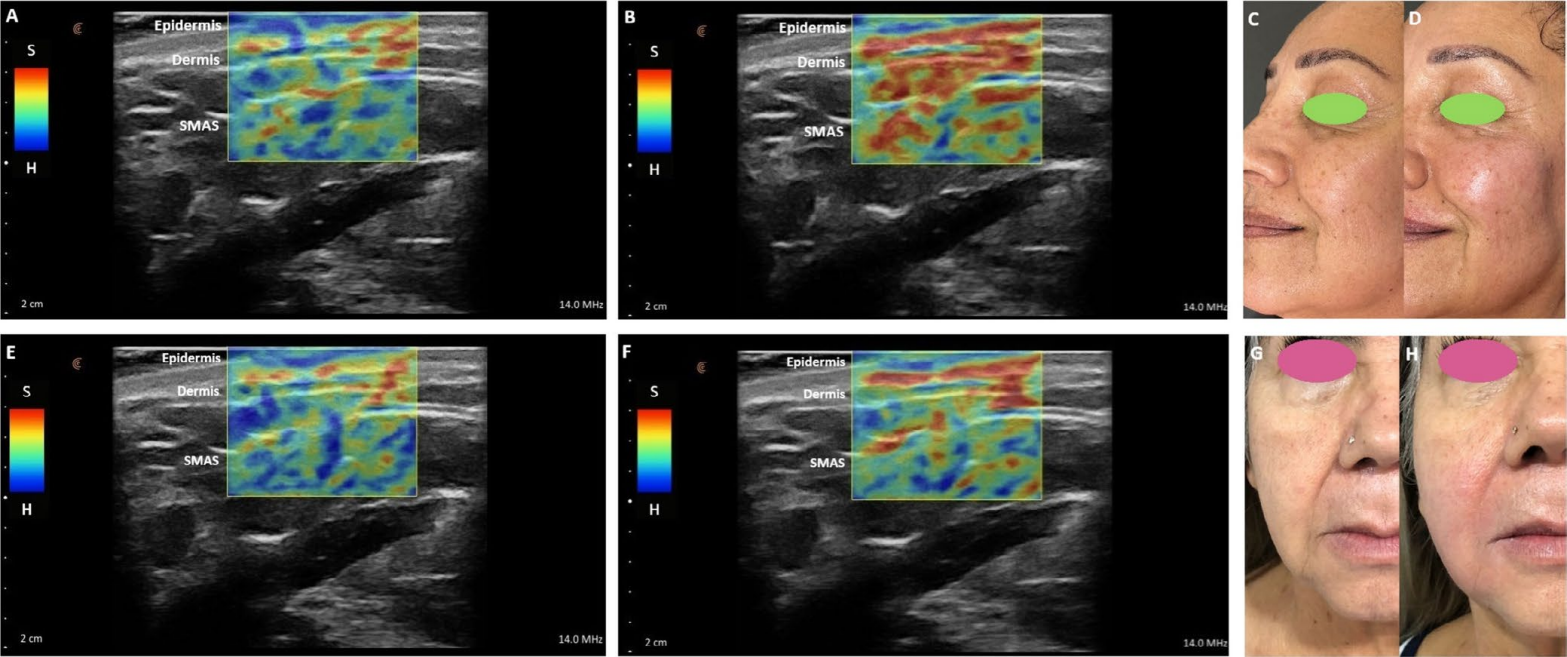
Ultrasound strain elastography and clinical images of two female patients before and after treatment with CPM‐R via the SkinGlow technique, showing improvement in tissue elasticity and firmness. Panels A‐D correspond to a 55‐year‐old female patient who received one session of the SkinGlow technique, with skin hydration increasing from 36.3% to 51.5%. Elastography images at baseline (A) and 30 post‐treatment (B); clinical image before (C) and after treatment (D). Panels E–H correspond to a 60‐year‐old female patient who received 2 sessions of the SkinGlow technique, with skin hydration increasing from 22.9% (baseline) to 32.0% (D30), and subsequently to 39.6% (D60). Elastography images at baseline (E) and D60 post‐treatment (F); clinical image before (G) and after treatment (H). In the elastograms, the grayscale displays the anatomical structures, while the colored overlays represent tissue elasticity through strain elastography. S (Soft): Areas with greater deformation (softer tissue), shown in warm colors (red, orange, and yellow), H (Hard): Areas with minimal deformation (stiffer tissue), shown in cool colors (green and blue).
